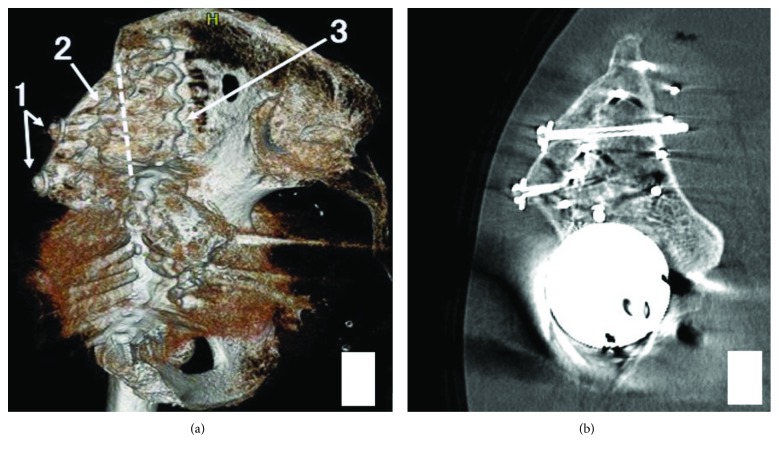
Reconstructed computed tomographic image (a). The dotted line indicates the osteotomy line of the iliac bone. The slid bone is fixed by two screws (1) and a small plate (2), and (3) indicates the reconstruction plate. Cross-sectional computed tomographic image (b).
